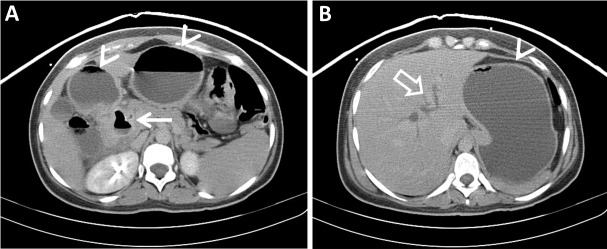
Contrast-enhanced Axial CT of the Upper Abdomen (Pre-Treatment) Contrast-enhanced axial CT images of the upper abdomen demonstrate (A) a periampullary duodenal diverticulum with surrounding inflammatory changes consistent with diverticulitis, including wall thickening and fat stranding (arrow). (B) An axial slice slightly more cephalad shows dilated intrahepatic bile ducts (open arrow). Also seen are the stomach and proximal duodenum, which are dilated with air-fluid levels (arrowheads).
